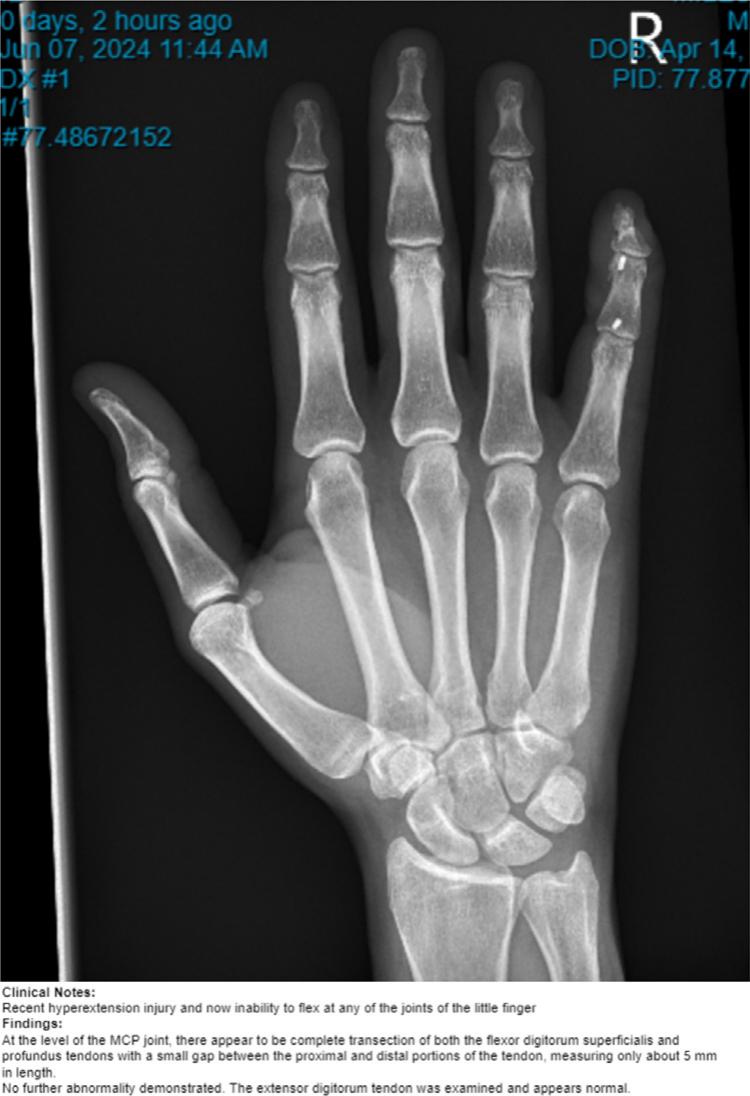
Frontal X-Ray of right hand (Case 1).
